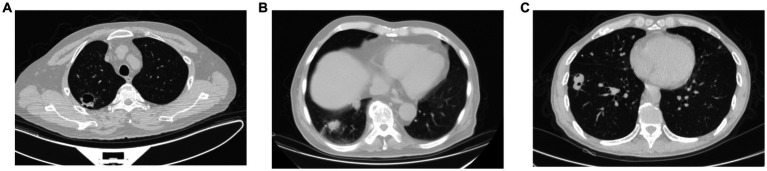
Chest CT in the lung window showing different pulmonary radiographic abnormalities. (A) Spiculation presented around pulmonary lesion. (B) A cavity formed within the nodule-shaped lesion. (C) Halo sign presented around the pulmonary lesion.
